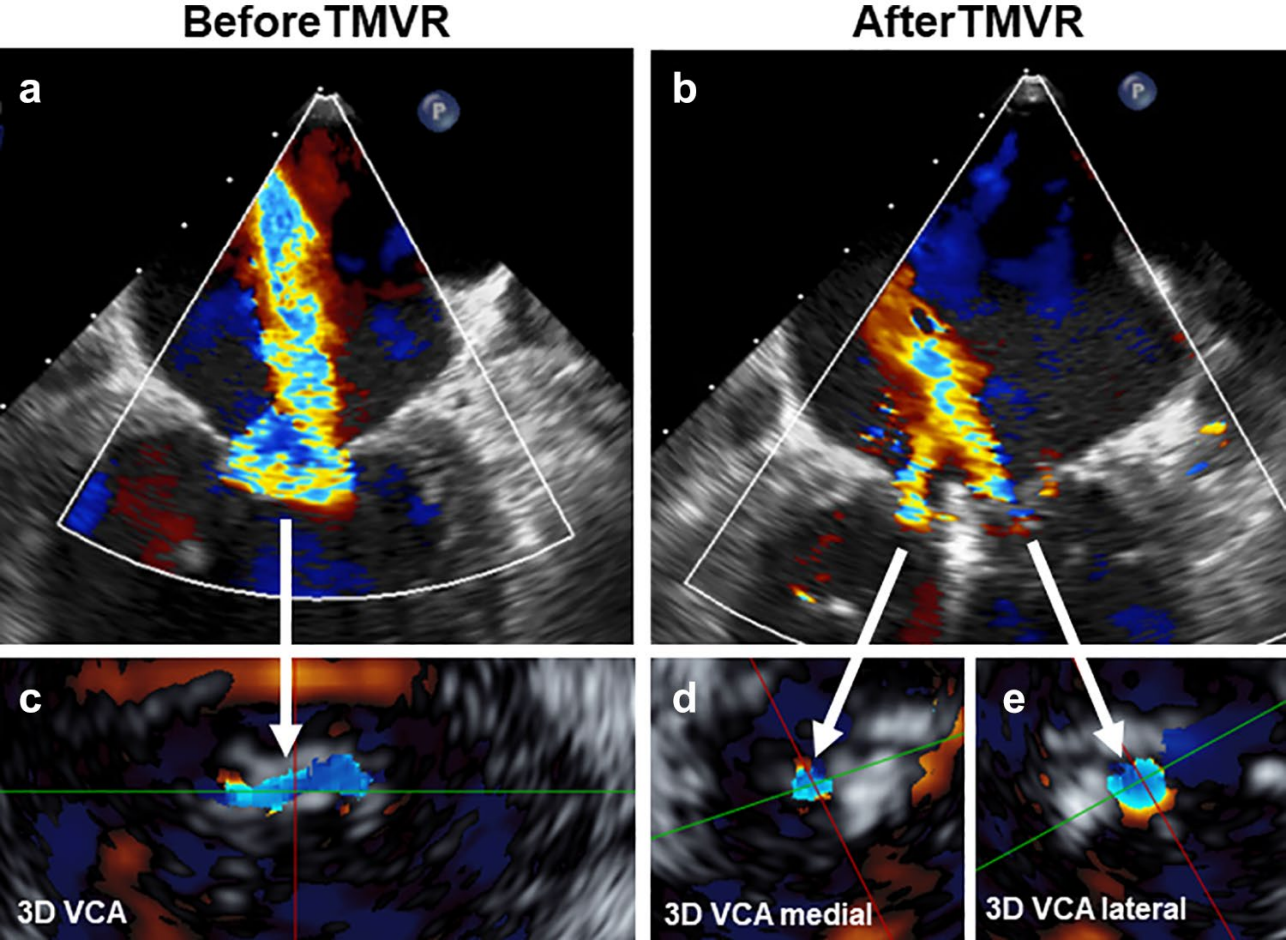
Assessment of 3D Vena contracta area (VCA). 2-dimensional TEE color flow Doppler intercommissural view before TMVR (a) and after deployment of one MitraClip XTR device (b). 3D TEE color Doppler with cut plane orthogonal to the regurgitant jet allows direct visualization of VCA before TMVR (c). After TMVR, 3D VCA was assessed separately for the remaining regurgitation jet located medial (d) and lateral to the clip (e). Total VCA was obtained by summation of both areas. *3D* 3-dimensional, *TEE* transesophageal echocardiography, *TMVR* transcatheter mitral valve repair, *VCA* vena contracta area
